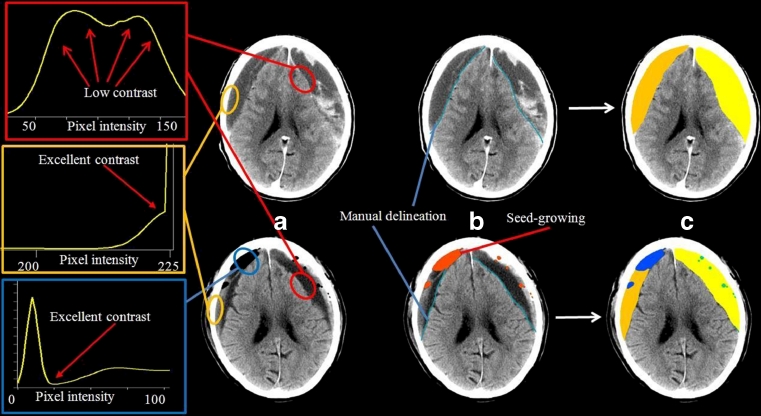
The segmentation and annotation of fluid and air volumes in preoperative and postoperative CT scans. *Upper row*: preoperative images. *Lower row*: postoperative images. Curves to the far left show typical intensity histograms for important image contrasts: brain/haematoma fluid/residual rinsing fluid (*red rectangle*); skull/all other tissues (*orange rectangle*); air/all other tissues (*blue rectangle*). a Representative preoperative and postoperative image sets; b manual delineation (*blue line*) and semi-automatic seed-growing of air pockets (*red fill*); c appearance after region-growing-based fill-in to generate areas with fluid and air, left and right
